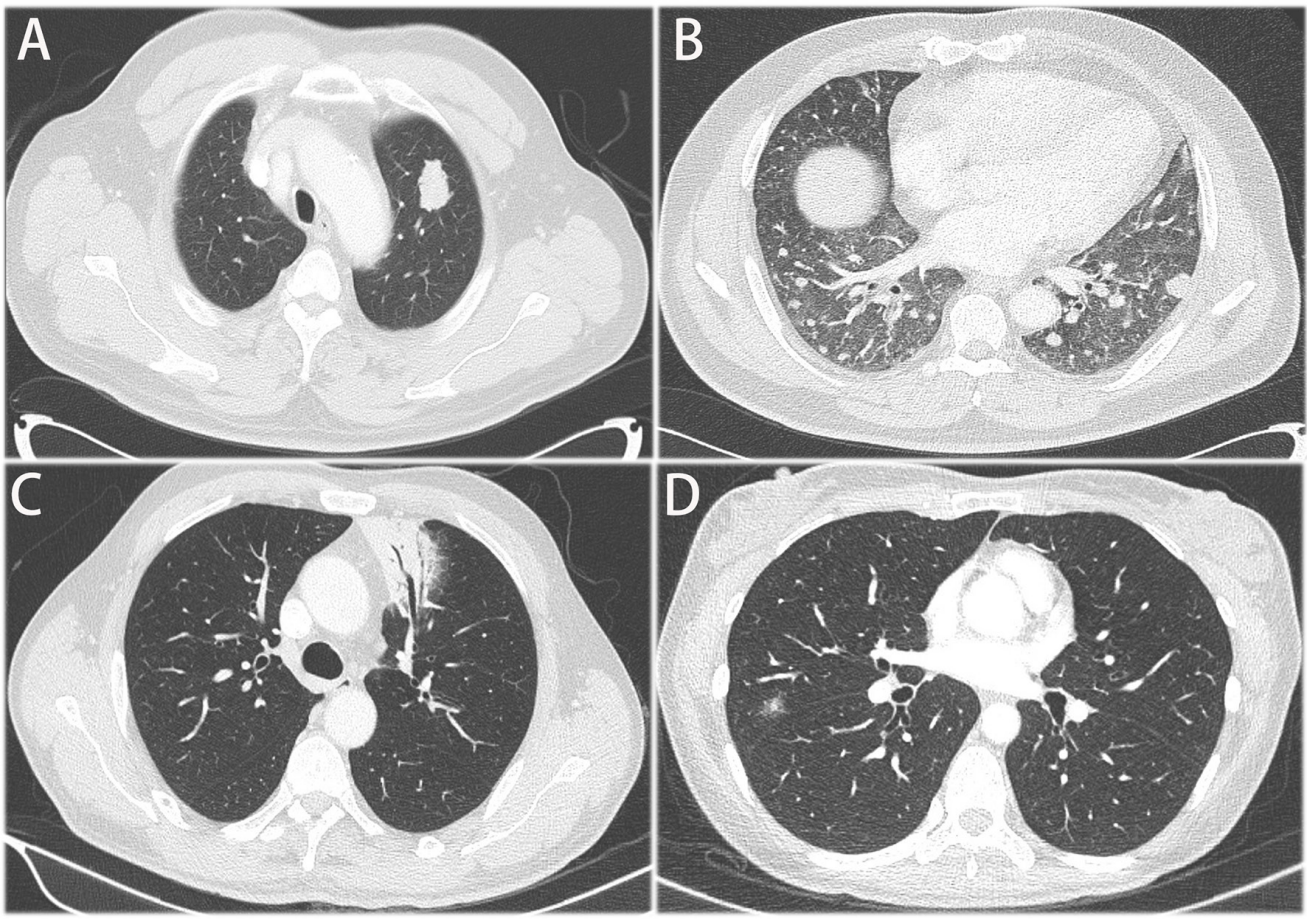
Representative CT images of primary pulmonary. (A) solid nodule or mass, (B) multiple nodules or masses (C) pneumonia-like consolidative pattern, (D) ground glass opacity.
